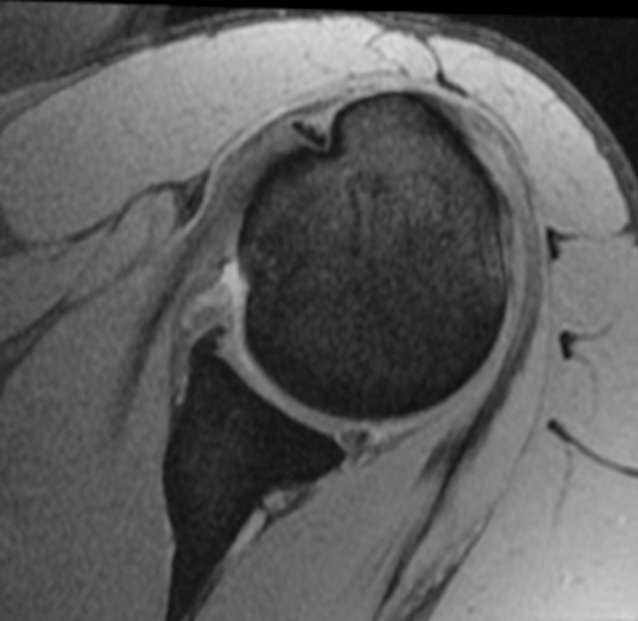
First-time posterior subluxation (A1). Magnetic resonance image of a young male patient who sustained an acute posterior shoulder subluxation with small reverse Hill–Sachs lesion and minor posterior capsulolabral damage during sports participation without major trauma
